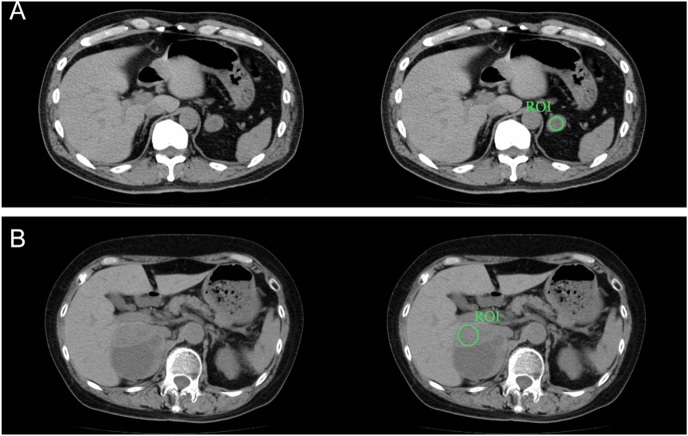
Simple rules for placing the region of interest (ROI). (A) In the case of a mass with a homogeneous interior, a circular ROI is placed to include the widest possible area of the mass. (B) In masses with a cystic component, the ROI is placed so that the largest circle can be drawn in the area without the cystic component. The same process is applied for calcification. Alt text: axial CT images showing methods for placing regions of interest (ROIs). (A) ROI placed to encompass the widest possible area within a homogeneous mass. (B) ROI placed to exclude cystic components and calcifications.
